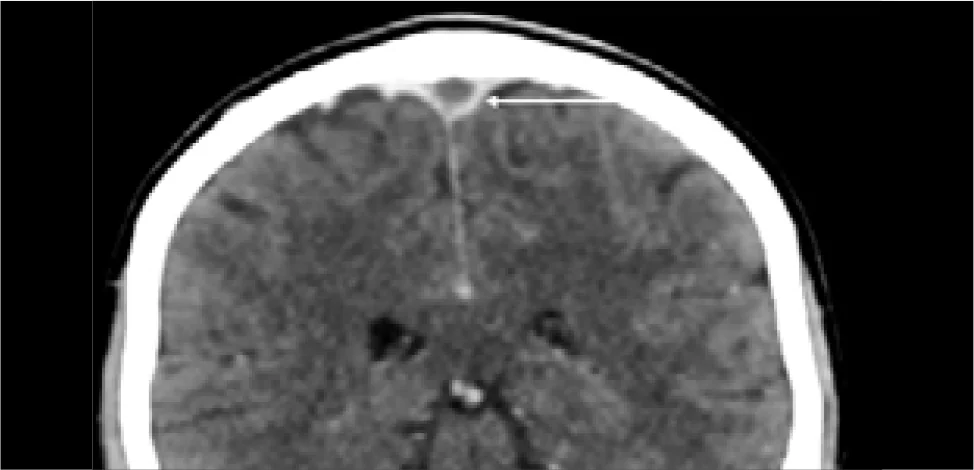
CT of a 41-year-old woman presenting with headache. Coronal imaging
